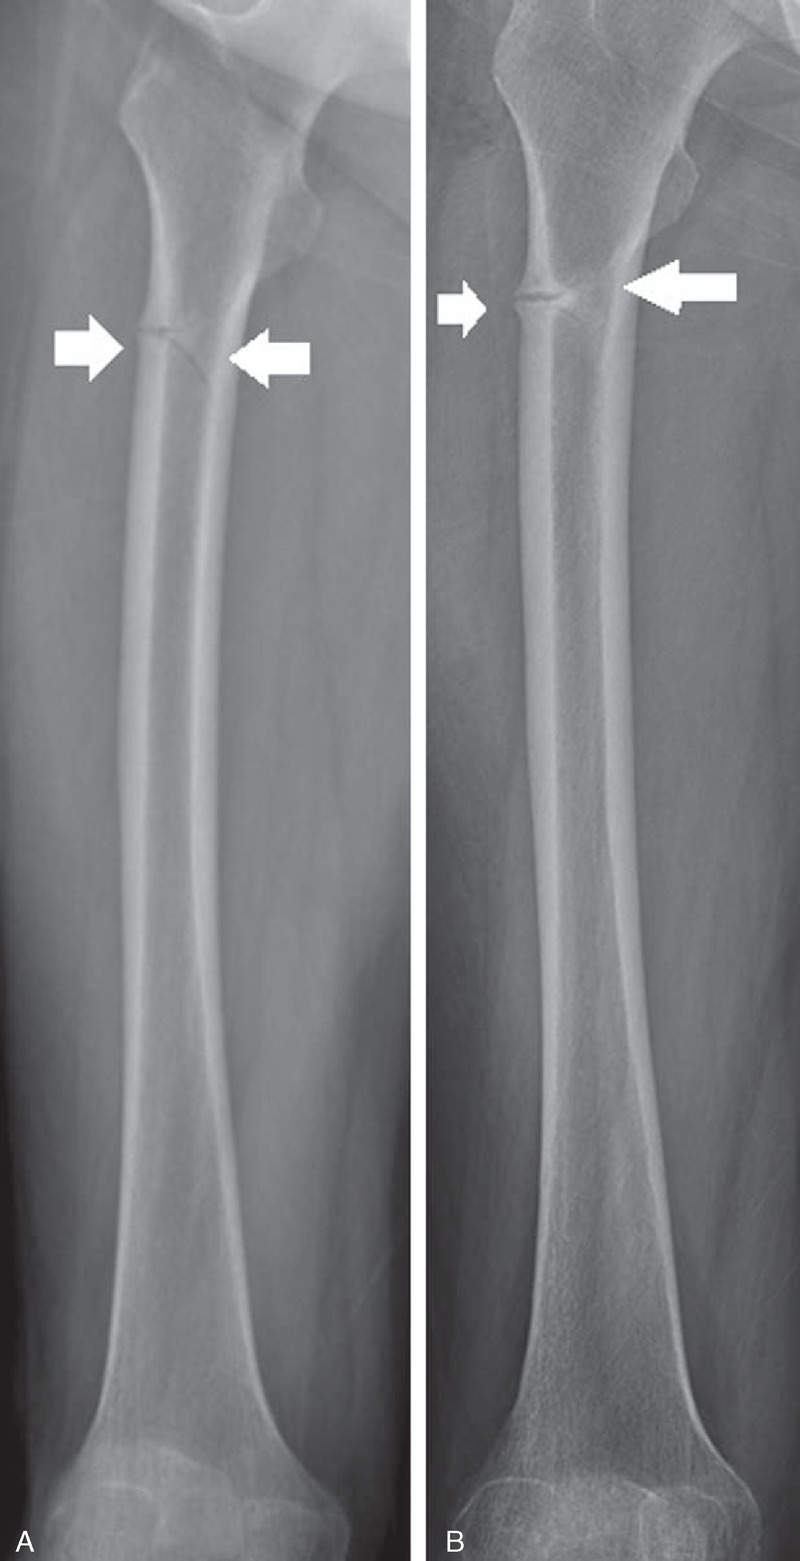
(A) Femur x-ray was conducted at the time of diagnosis (arrows). (B) Femur x-ray was conducted after conservative management (arrows).
